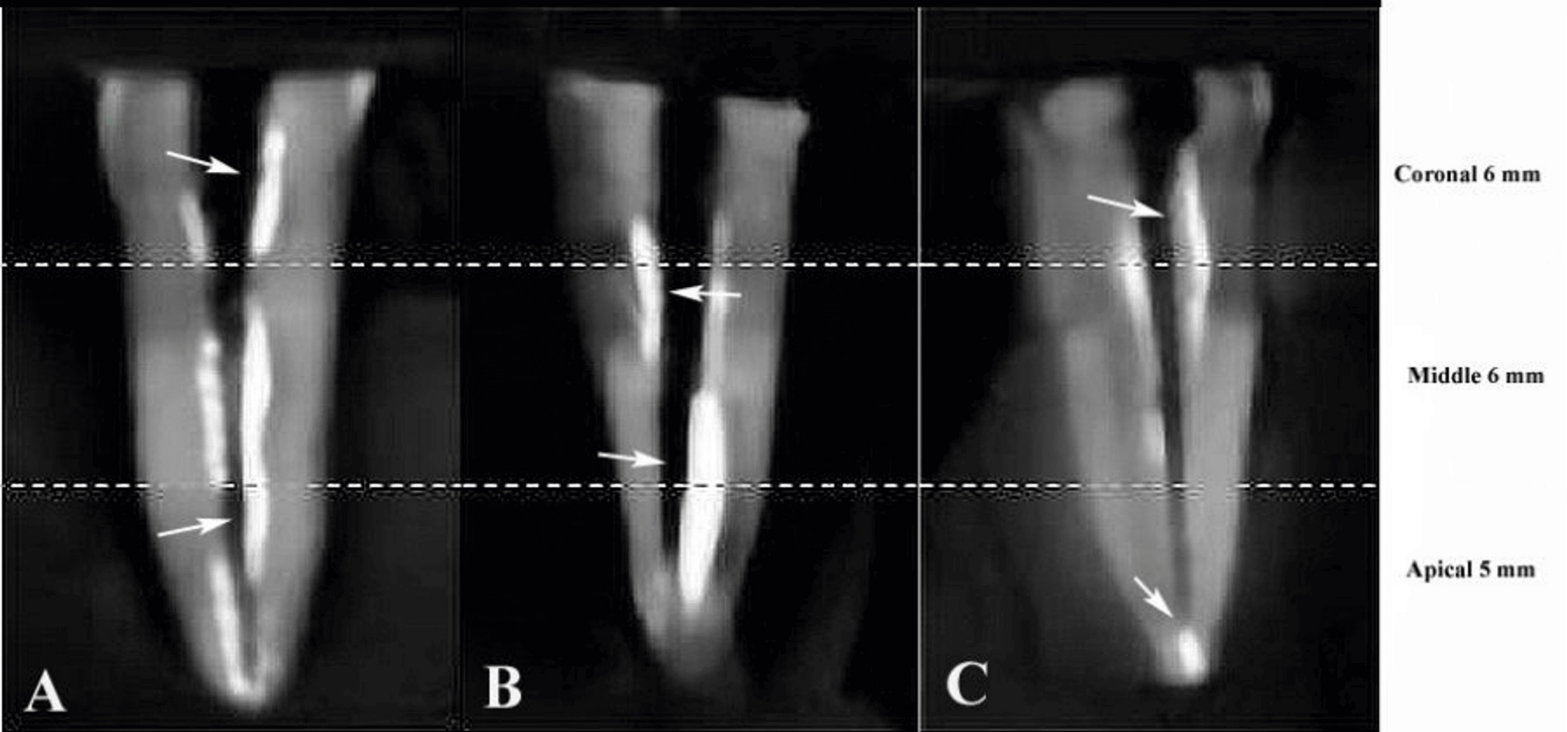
Remnants of sealer on dentinal wall shown by cone-beam computed tomography (CBCT) images of root canal at various levels. (A) AH Plus. (B) Sealmax-R. (C) Epoxidin. Original CBCT images of the teeth in the study.
